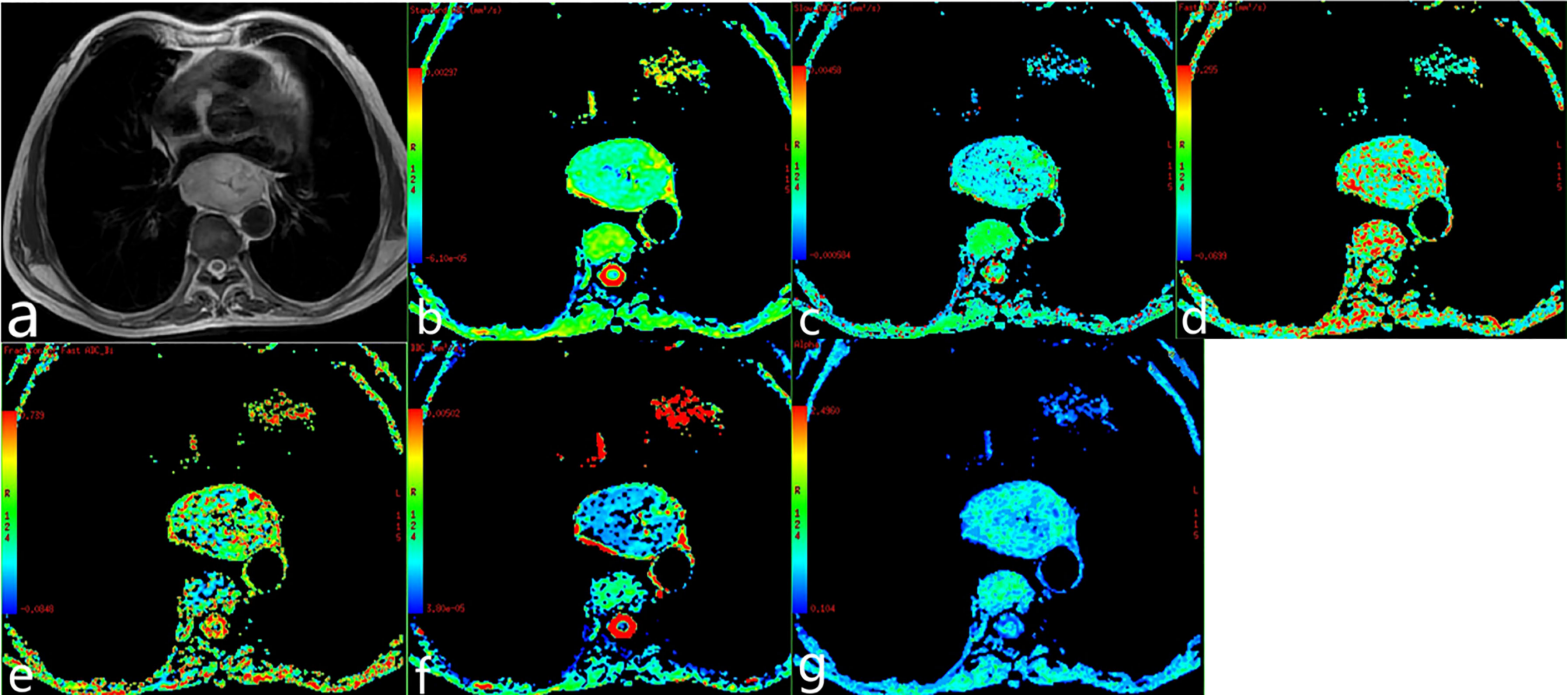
Poorly differentiated esophageal carcinoma of a 62-year-old man. (A) T2-weighted image. (B–G) ADC map, ADC_slow_ map, ADC_fast_ map, *f* map, DDC map, and α map.
